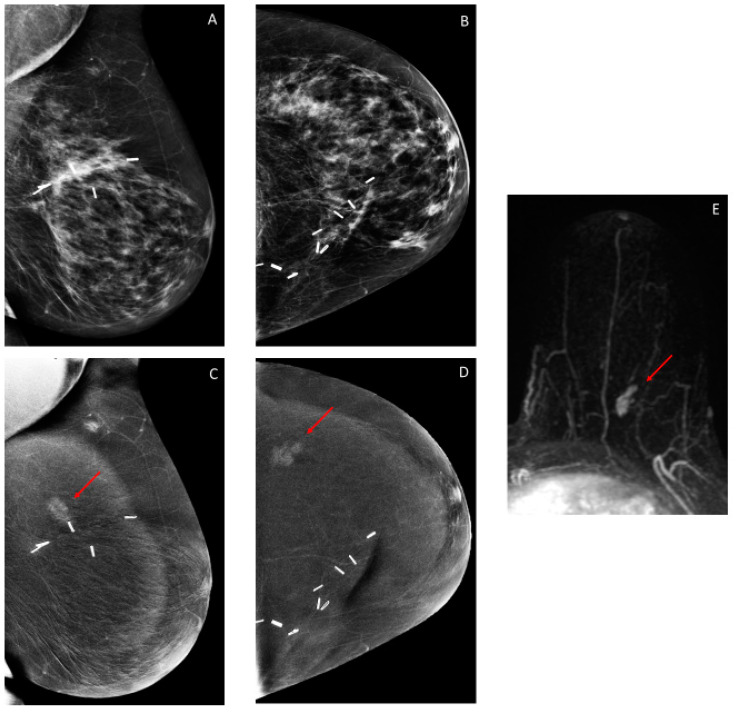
Sixty-five-year-old woman treated with left breast conservation surgery 17 years prior for invasive ductal carcinoma (IDC) and ductal carcinoma in situ (DCIS). Screening CEM shows no suspicious findings on the low-energy images: (A) left mediolateral oblique (MLO) and (B) left craniocaudal (CC). Iodine images: (C) left MLO and (D) left CC show focal enhancement in the upper outer quadrant (arrows), new from the prior CEM exam. Targeted ultrasound showed no sonographic correlates. Breast MRI showed correlative enhancement ((E) left breast subtraction maximum intensity projection (MIP)); the lesion was biopsied with MRI guidance, yielding DCIS. Left lumpectomy was performed, yielding DCIS. Stable-enhancing skin lesion noted superiorly on MLO images and laterally on subtraction MIP.
